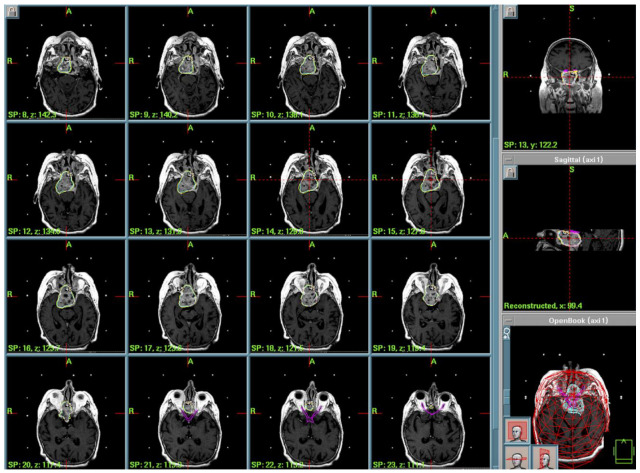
Treatment planning. Volumetric T1-weighted MRI. In yellow is the prescription isodose of the large skull base lesion. In purple is the contouring of the anterior optic pathways.
